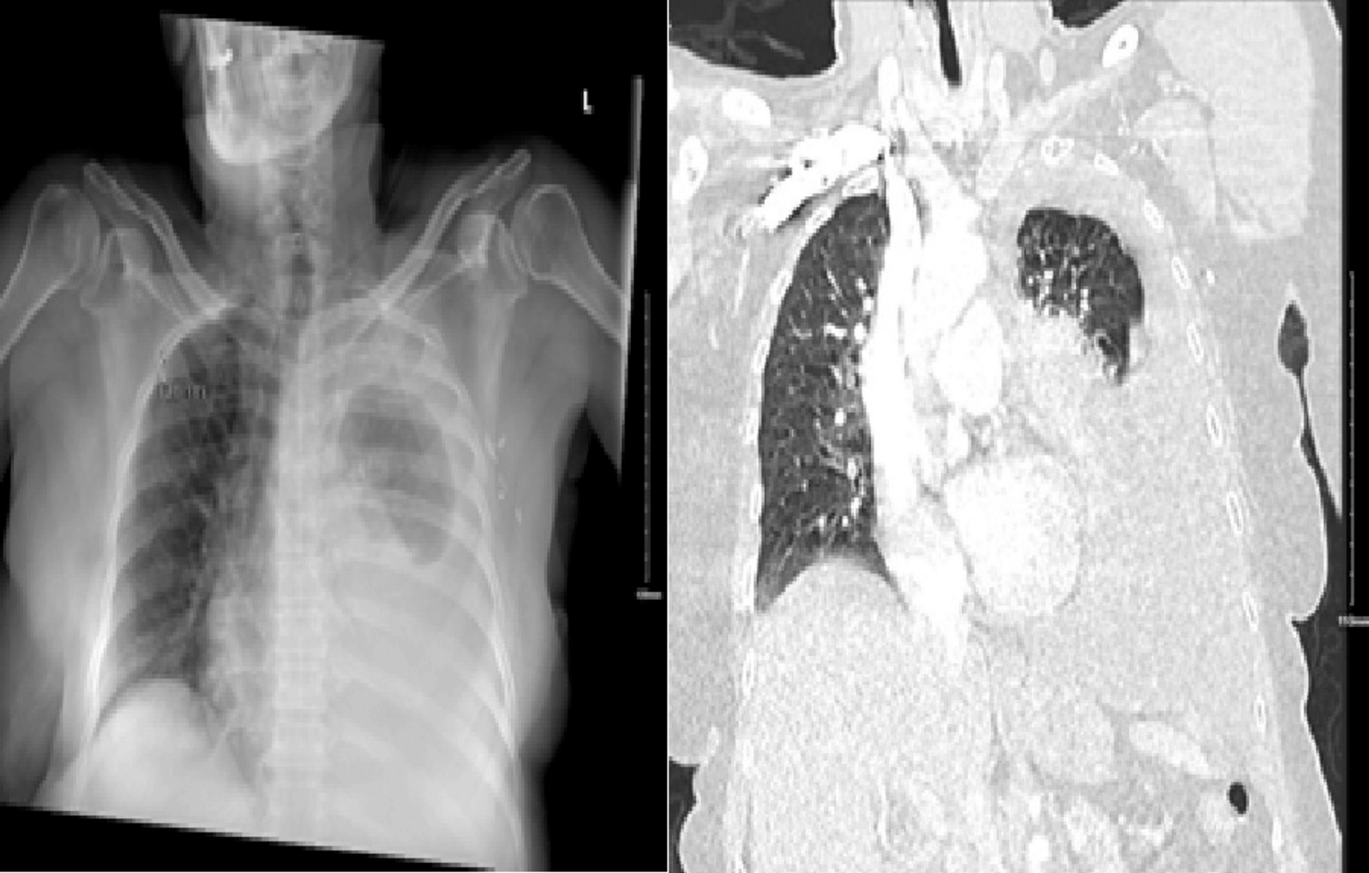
Chest X-ray and chest CT (lung view) demonstrating a large left-sided pleural effusion
